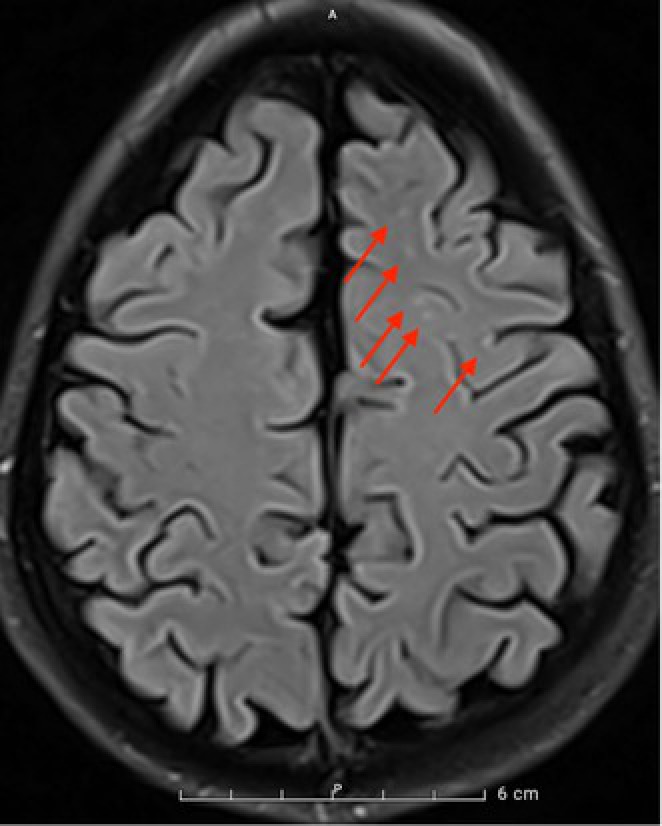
FIGURE 1 30 years‐old. FLAIR. Small lesions adjacent to the left frontal cortex.
